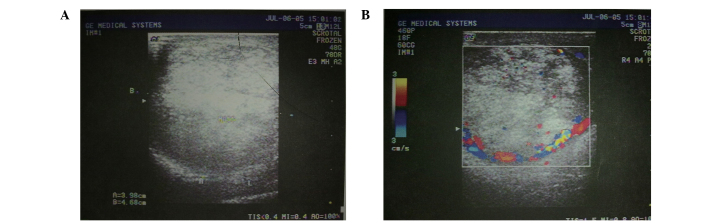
(A) Ultrasonography of the mass in the left scrotum shows a medium echo solid mass with heterogeneous echo texture. (B) Color Doppler image shows vascularity inside and around the mass.
